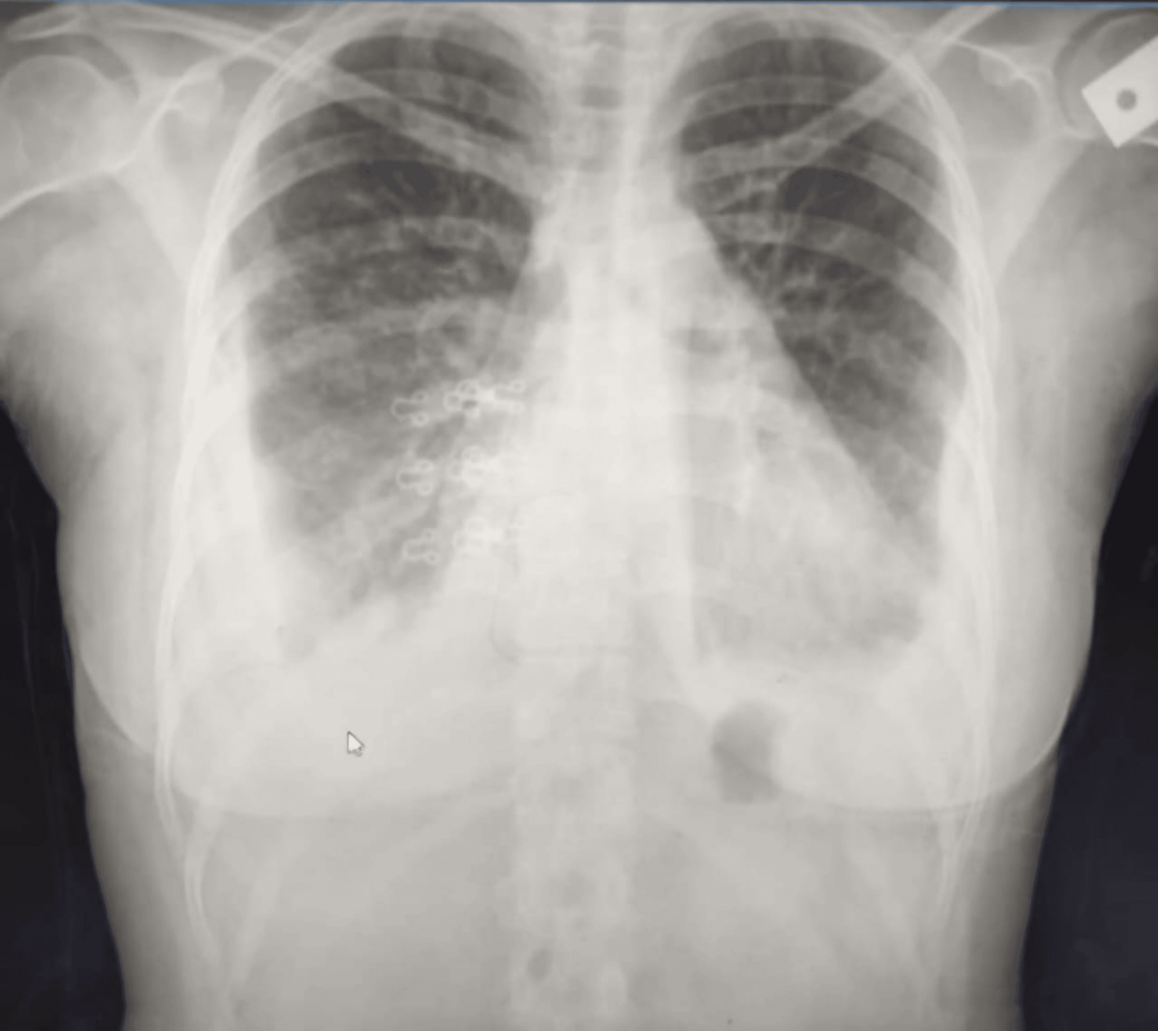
Chest X-ray revealing bilateral pleural effusion with pulmonary infiltrates
